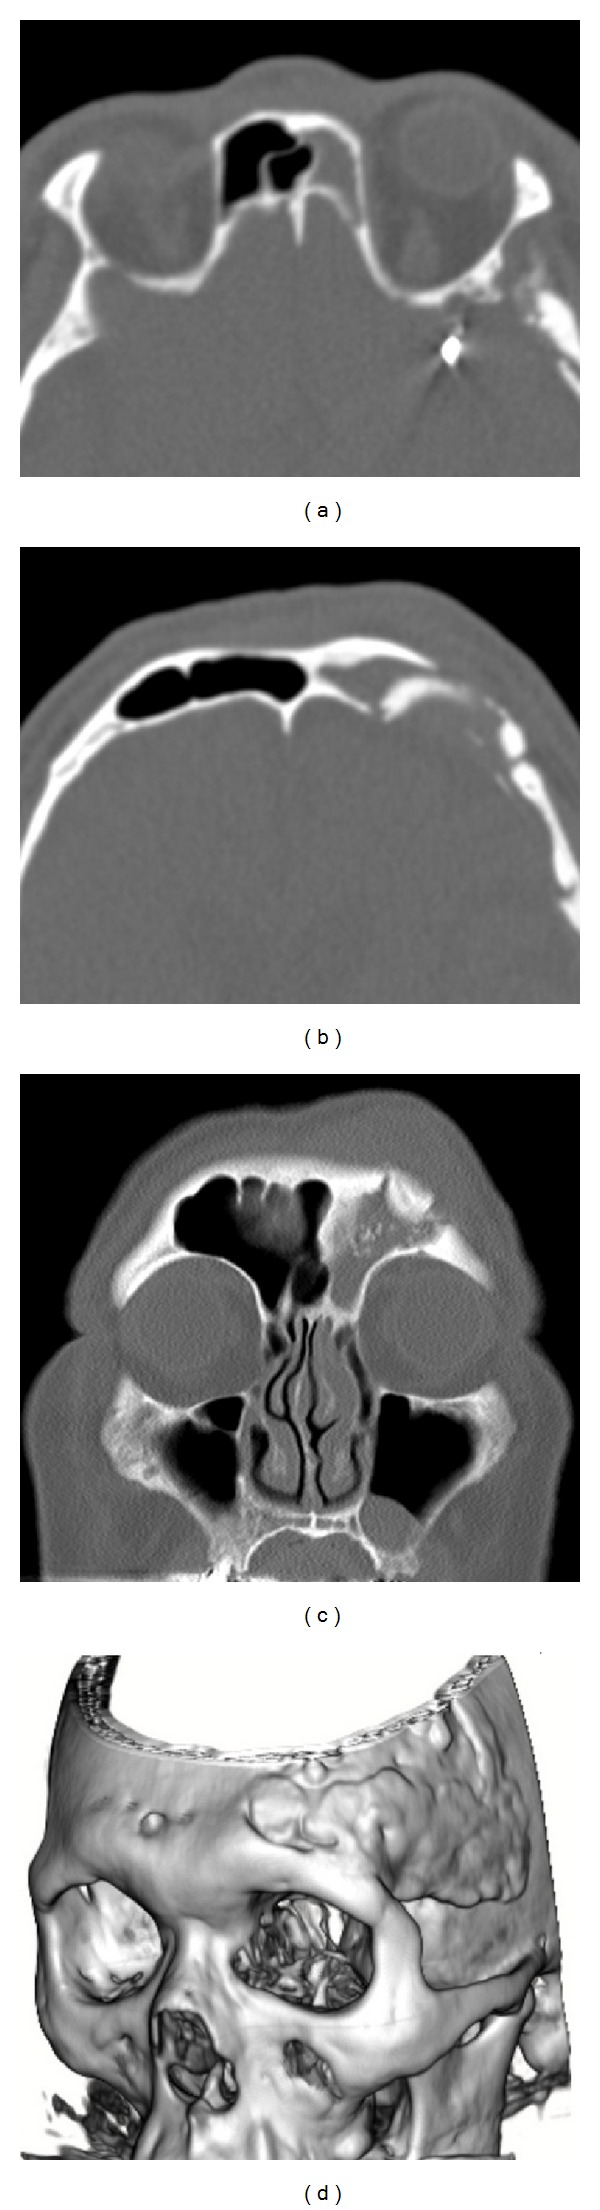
Preoperative computed tomography scans. (a) Axial scan. Left frontal sinus is opacified. Intracranial clip is seen. (b) Axial scan. Frontal bone is deformed as a result of previous neurosurgery. Left frontal sinus has corners with acute angles. (c) Coronal scan. High-density spot is seen in the left frontal sinus. The corners of the frontal sinus are acute. (d) Three-dimensional reconstruction. Postoperative change is seen in the left frontal bone.
